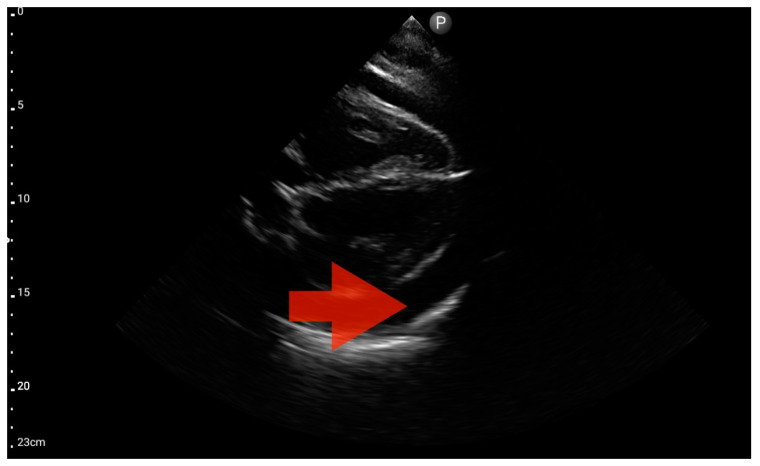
Pericardial effusion; position: apical four-chamber (FATE protocol). Sector probe; the red arrow indicates free fluid in the pericardium, which did not cause a sonographic image of cardiac tamponade (source: author’s material—DK).
